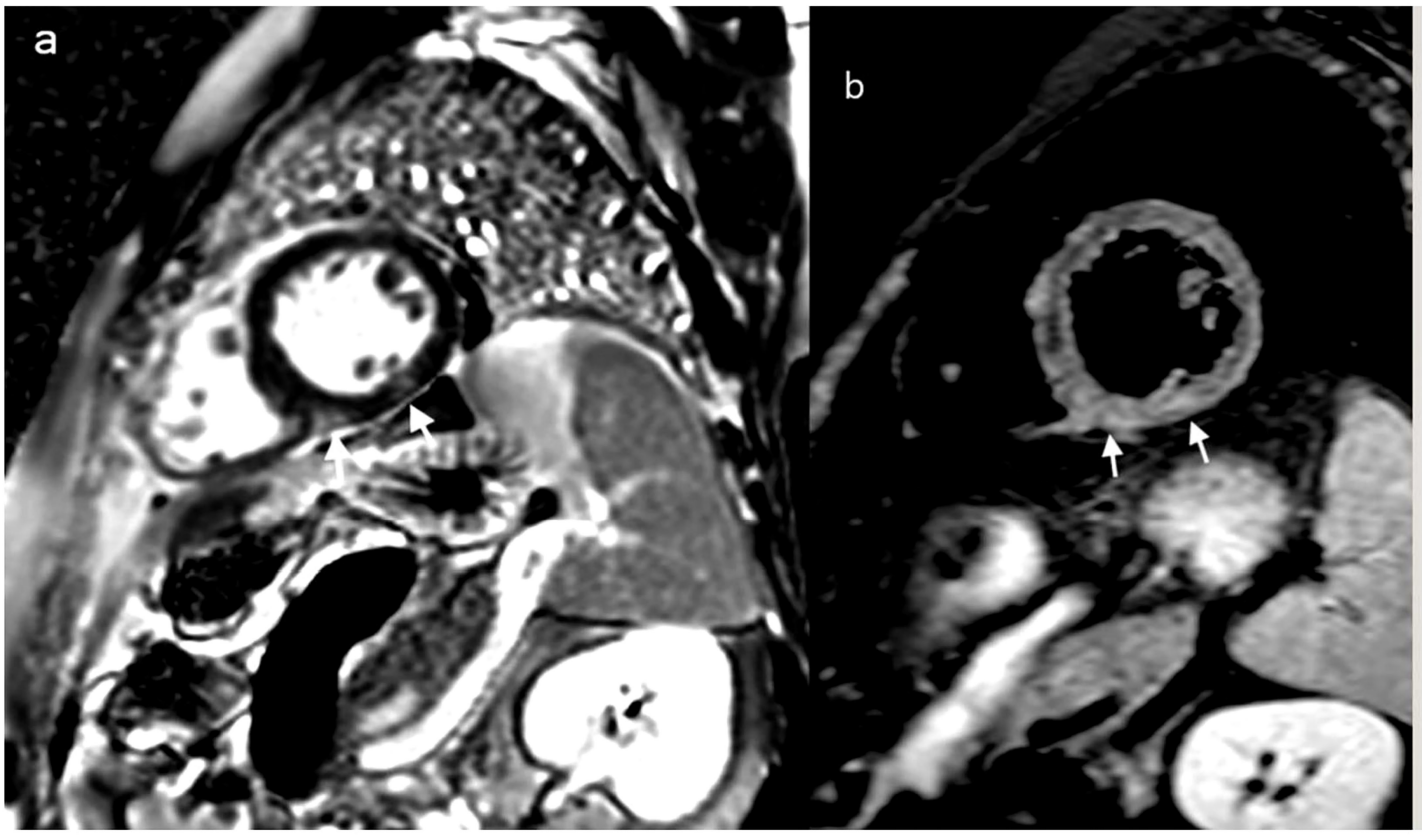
Cardiac MRI: delayed MRI enhancement sequence using the short-axis (A) highlights two contrast upateks with mid-myocardial and sub-epicardial topography at the level of the infero-septal and inferior wall of the left ventricle (white arrows) which correspond to focal ares of mycocardial edema visible in T2 weighted image with fats saturation (B) white arrows, suggesting acute myocarditis.
